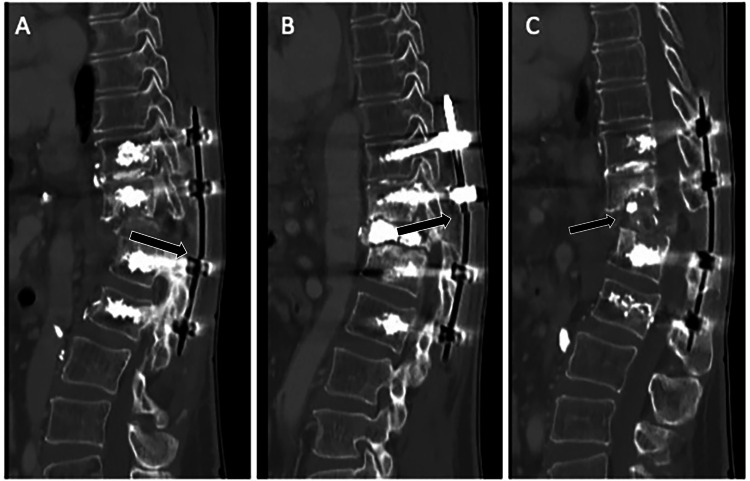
Preoperative imaging. CT demonstrating bilateral broken rods (A) and (B) and subsidence of the T12 corpectomy cage (C). CT: computed tomography.
